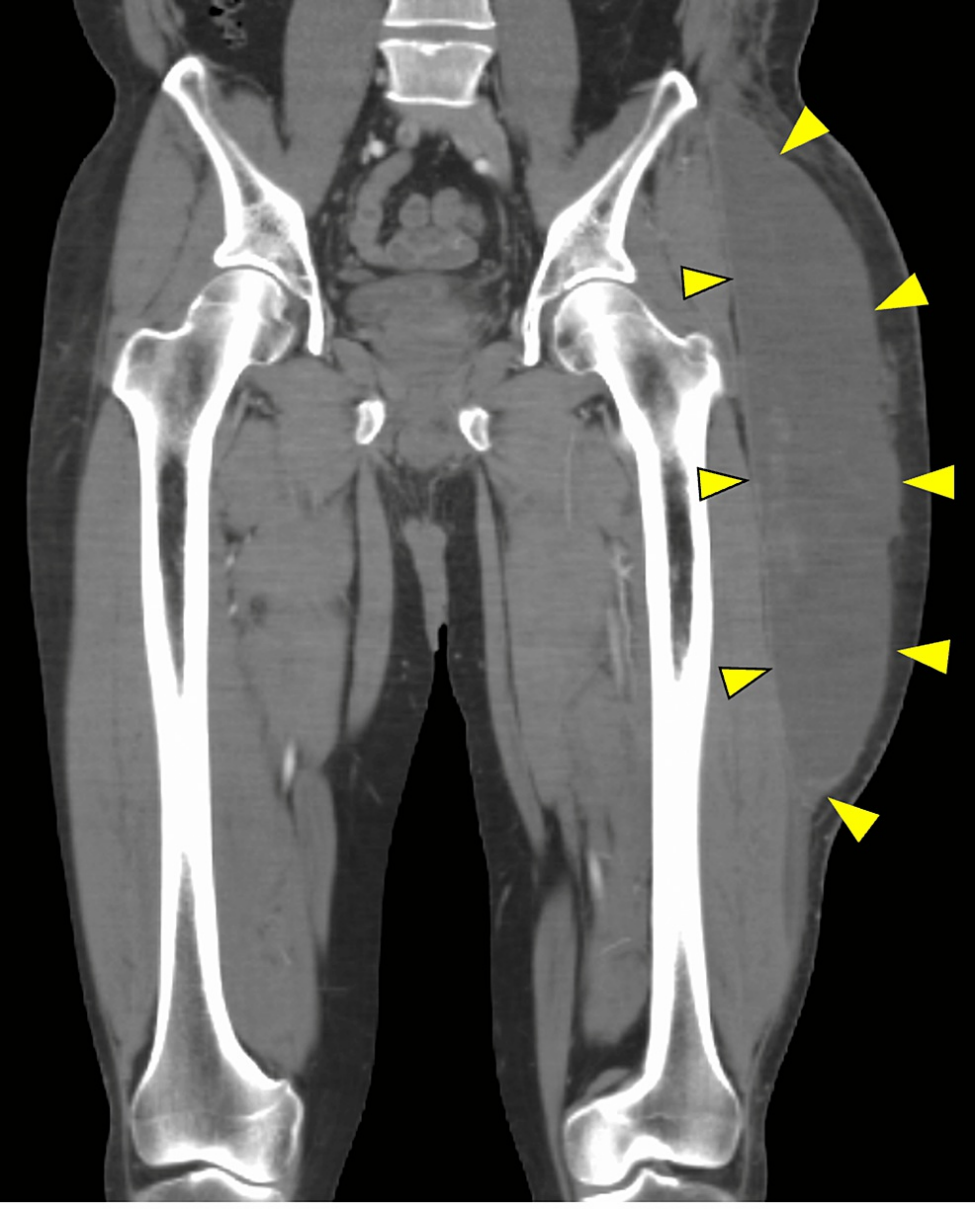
A CT image coronal plane A hematoma-like effusion was observed on the lateral aspect of the iliotibial band (yellow arrowheads)
